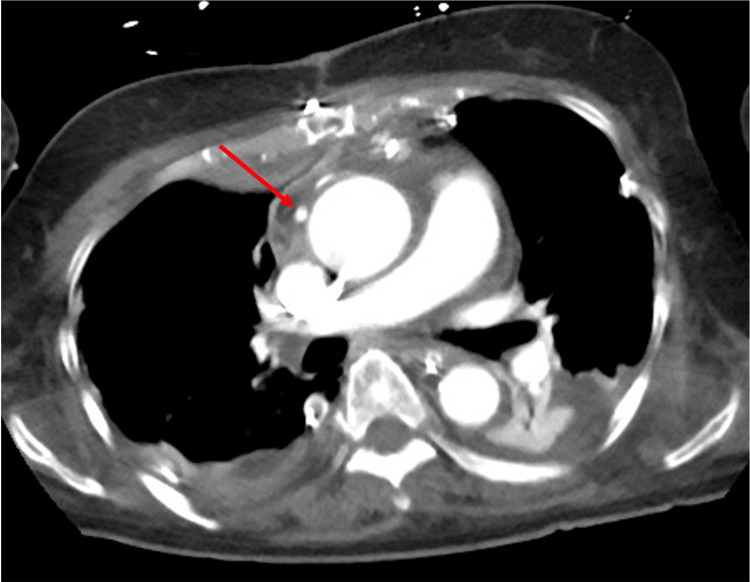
Postoperative contrast-enhanced computed tomography The red arrow indicates saphenous vein graft and the bypass to the right coronary artery was patent.
